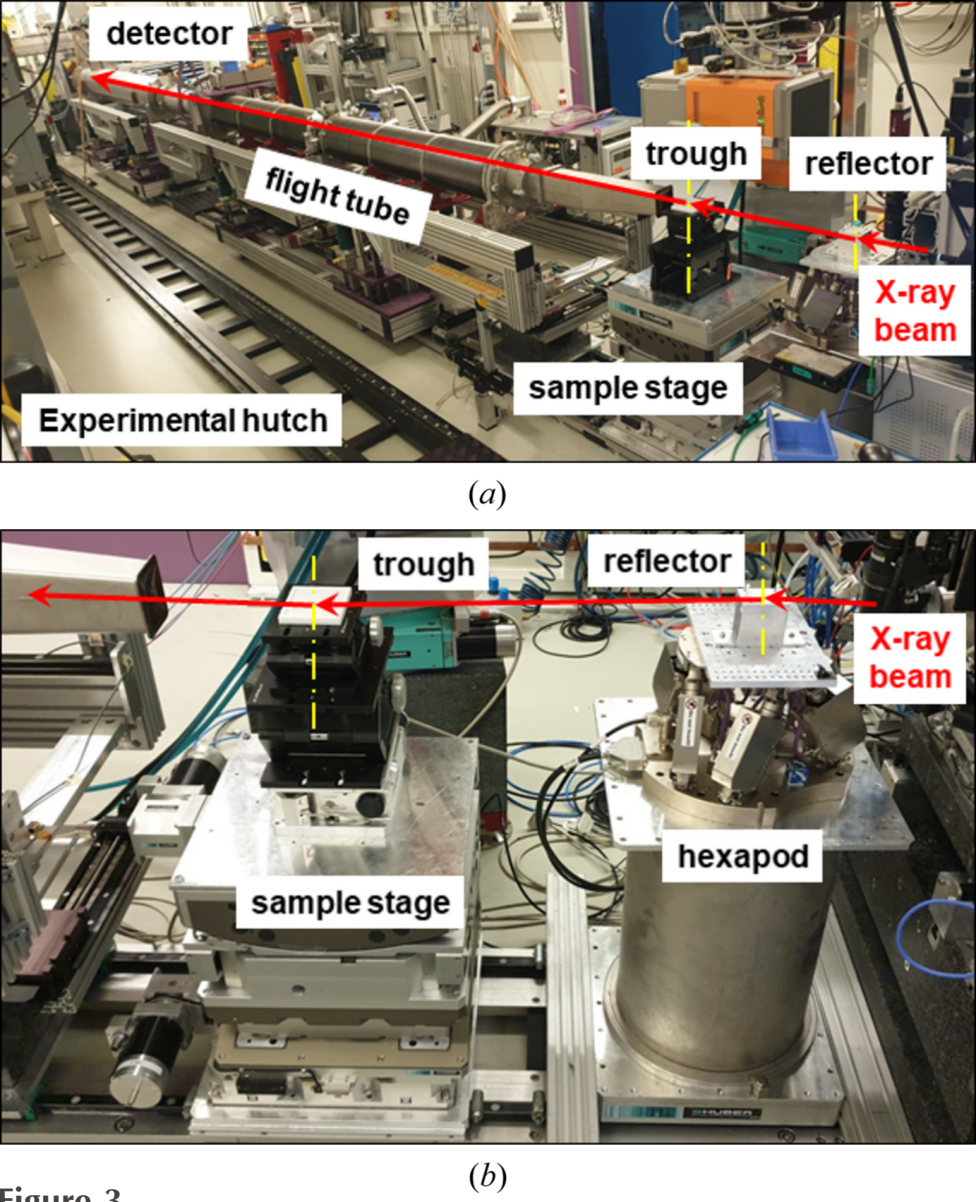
(*a*) General view of the setup with the realized scheme of tilting the X-ray beam onto a liquid surface for the USAXS study inside the experimental hutch EH1 of the P03 station at the PETRA III synchrotron (DESY, Hamburg, Germany). (*b*) The reflector assembly was placed on the hexapod and the sample assembly was placed on the Huber sample stage (side view). Propagation directions of a collimated monochromatic X-ray beam focused on the reflector. The vertical axes of the reflector and the sample are shown by yellow dashed-dotted lines.
